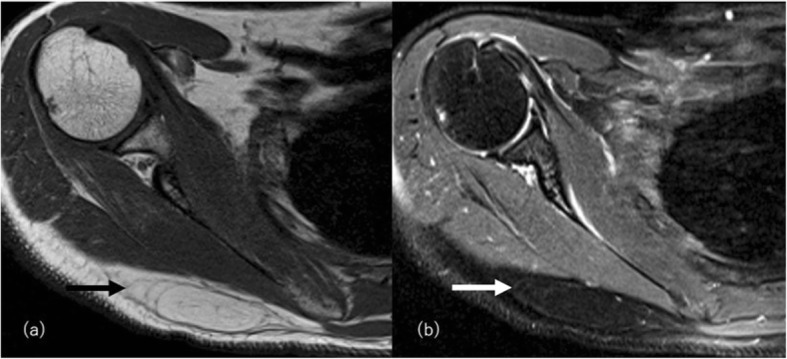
Superficial lipoma in a 60-year-old woman on the right shoulder. a T1-weighted image and (b) fat-suppressed T2-weighted show a homogeneous fatty mass (arrows) with a similar signal intensity to that of the adjacent subcutaneous fat but with a thin capsule and thin internal septa
